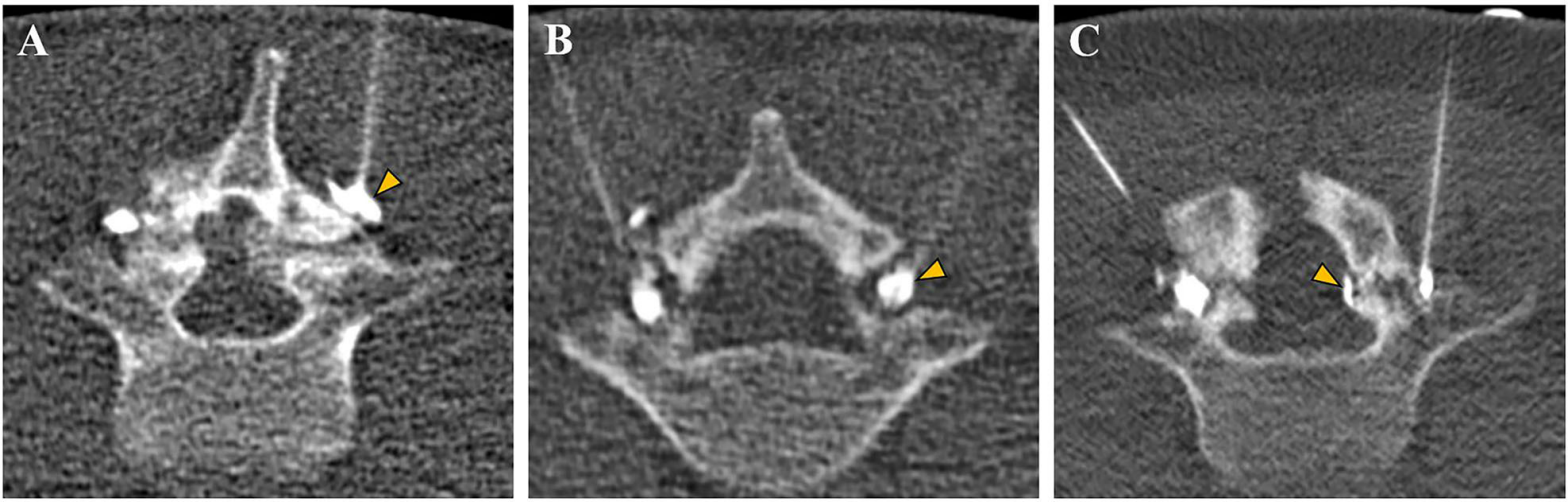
Three different contrast distribution patterns during CT-guided lumbar pars injections in three different patients. Contrast distribution within the soft tissues immediately surrounding the pars defect, coined peri-defect (yellow arrowhead), contrast distribution (A). Contrast distribution within the pars defect, coined intra-defect (yellow arrowhead), contrast distribution (B). If the contrast agent reached through the pars defect into the epidural space (yellow arrowhead), the pattern was coined trans-defect contrast distribution (C). In case different patterns were observed on each side, the deeper contrast distribution pattern was chosen for case classification. Thus, image (C) represents a case of trans-defect contrast distribution
